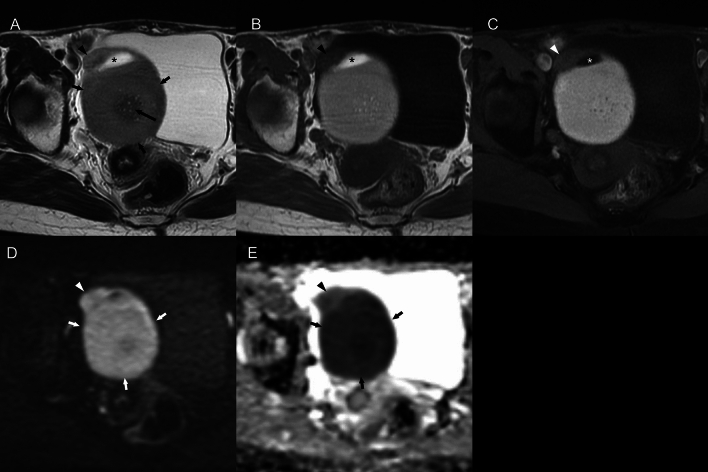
A 55-year-old woman with squamous cell carcinoma arising in an ovarian cystic teratoma without transmural extension, shows a mural nodule on MR images consistent with “findings suggestive of malignancy” (mural nodule with diffusion restriction and without fat content, calcification, or Palm tree appearance). a Axial fat-suppressed T2-weighted image reveals a 79 mm-sized cystic lesion in the pelvis. The mural nodule (arrowhead) protruded from of the ventral cyst wall does not exhibit a Palm tree appearance and forms an obtuse angle with the cystic wall. While the nodule also protrudes outward from the wall, its margins are smooth, and there are no findings suggestive of transmural invasion. There is a hyperintensity area adjacent to the mural nodule suggesting fat component (*). The dorsal cystic portion was shown as a slightly hyperintense area (short arrows). A hypointense area in the dorsal cystic portion suggests hair ball (long arrow). b, c Axial T1-weighted and fat-suppressed images do not show any distinct fatty signals in the nodule (arrowhead) though there is a fat component (*) adjacent to the mural nodule. The dorsal cystic portion was shown as a hyperintense area on T1-weighted images with/without fat-suppression (short arrows). d, e An axial diffusion-weighted images and an apparent diffusion coefficient (ADC) map indicate restricted diffusion within the nodule (arrowhead). Most of the part of the dorsal cystic portion is also shown as diffusion restriction, suggesting it contains the mixture of keratin and sebum (short arrows)
